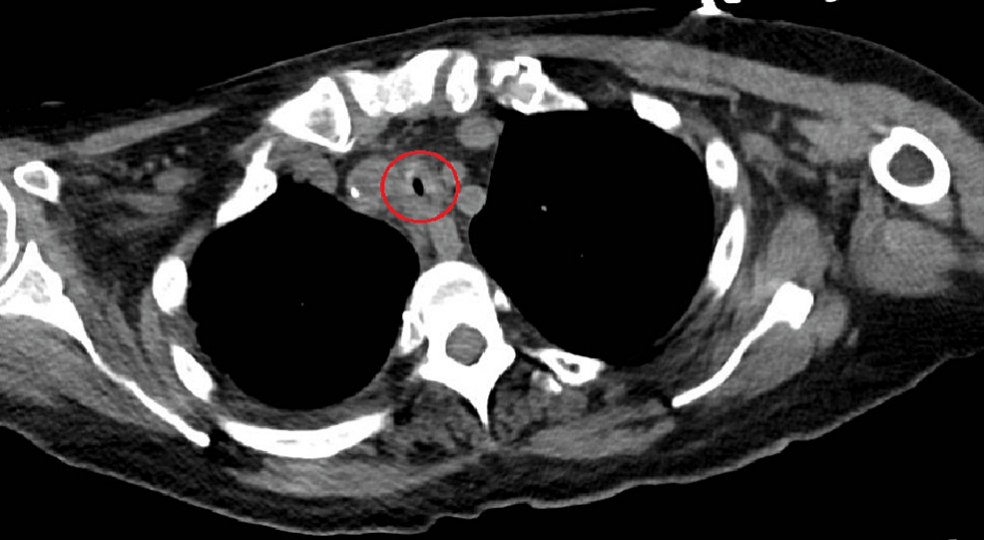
CT chest in axial view showing tracheal stenosis
